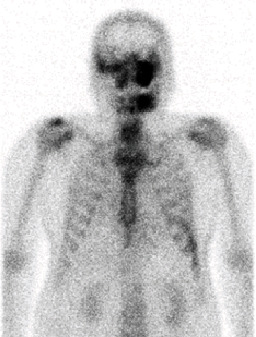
Bone scintigraphy findings obtained during the initial visit. Bone scintigraphy shows an accumulation of technetium medronic acid in the right skull base, cervical spine, left maxilla, and left mandible.
